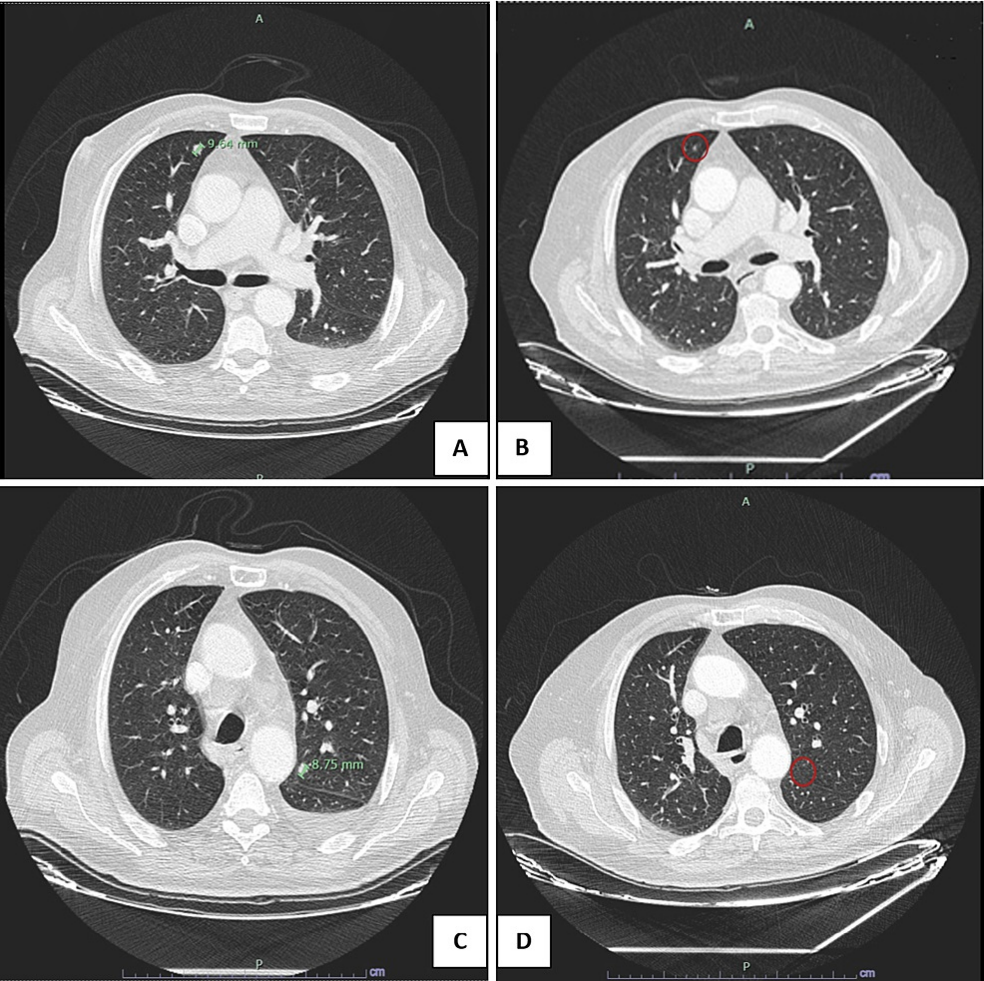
Thoracic CT (A and C) at avelumab discontinuation and (B and D) 10 months after discontinuation. (A and C) Multiple micronodules were visible at various locations with a maximum size of 8-9 mm when avelumab was discontinued. (B and D) The complete response was observed at 10 months with the disappearance of previously described micronodules.
